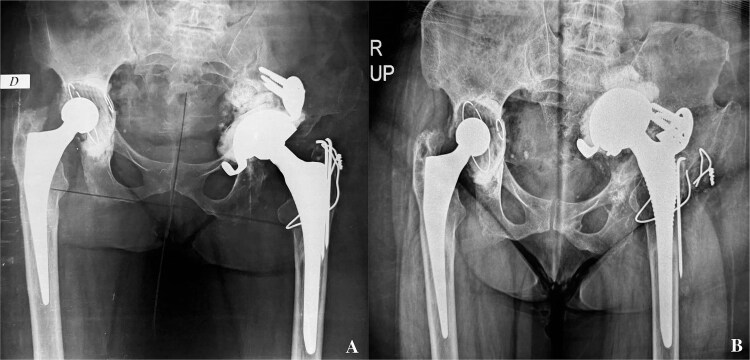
A- revision of the left total hip arthroplasty: postoperative pelvis X-ray. B- pelvis X-ray: Intrapelvic migration of acetabular components (Kerboul cross-plate and the cup).
